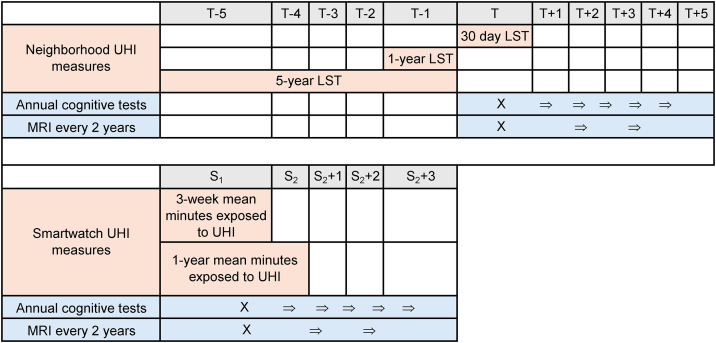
Timing of HBI UHI exposures and cognitive and MRI outcomes. The upper panel shows timing for neighborhood-level urban heat island (UHI) exposure measures derived from land surface temperature (LST) relative to the baseline HBI visit (T). Recent exposure is defined as the mean LST during the 30 days before T, and cumulative exposure is defined as mean LST during the 1-year and 5-year periods before T. Cognitive assessments are conducted annually beginning at T, and MRI is conducted at T and every two years thereafter. The lower panel shows timing for the smartwatch sub-study. Smartwatch-based UHI exposure is summarized as mean minutes exposed to UHI conditions during the first 3-week monitoring period (S1) and as the 1-year mean across the first and second smartwatch monitoring periods (S1 and S2). X indicates the index cognitive or MRI assessment, and arrows indicate follow-up assessments. Abbreviations: HBI, Healthy Brain Initiative; LST, land surface temperature; MRI, magnetic resonance imaging; S1, first smartwatch monitoring period; S2, second smartwatch monitoring period; T, baseline HBI visit; UHI, urban heat island.
